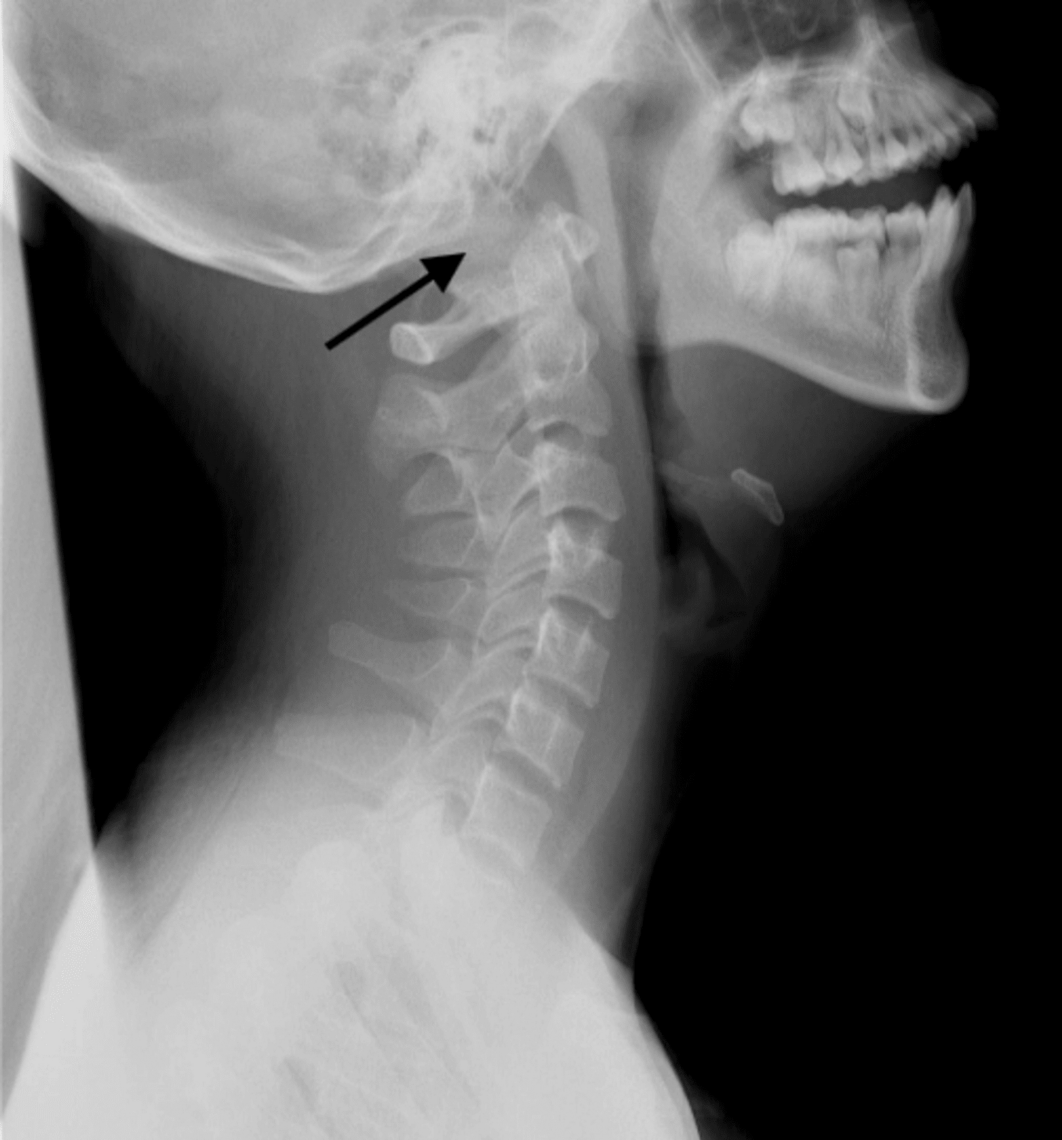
Preoperative lateral view of cervical x-ray for the patient. The x-ray showed subluxation of the atlantooccipital joint (arrow).
